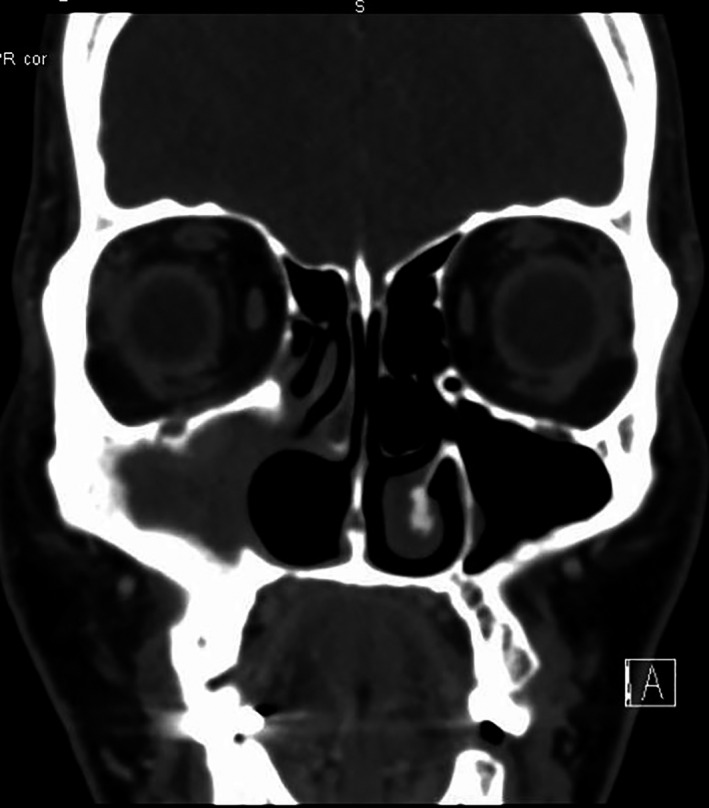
Sinus CT scan with contrast showing postoperative changes of uncinectomy and medial maxillectomy
